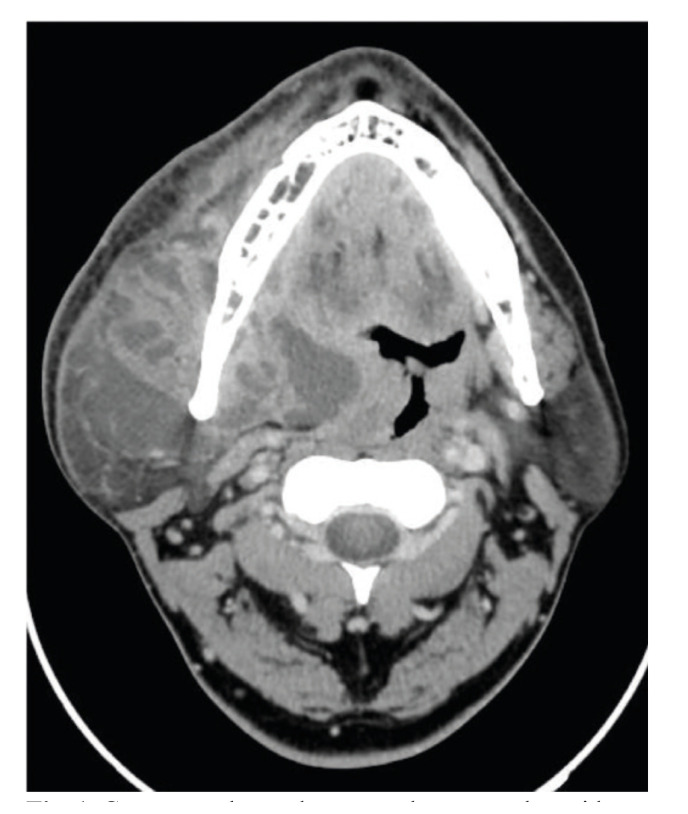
Contrast-enhanced computed tomography evidencing a multilocular abscess with extension to the submandibular, parapharyngeal, buccal, and masticator neck spaces.
